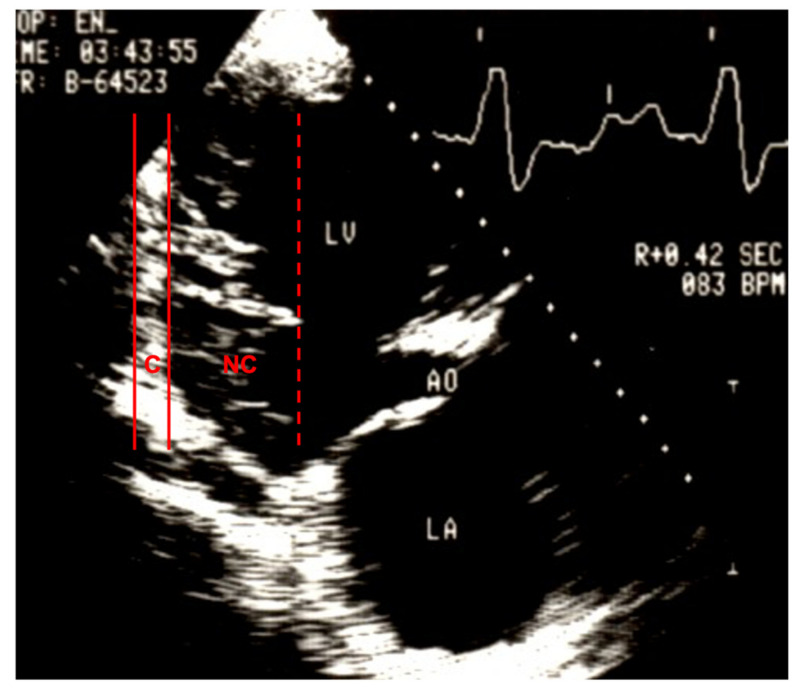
Echocardiography of the first published patient with NCCM without congenital heart disease. LV—left ventricle; LA—left atrium; Ao—aorta; C—compacted layer; NC—noncompacted layer of the left ventricular wall with deep recesses.
